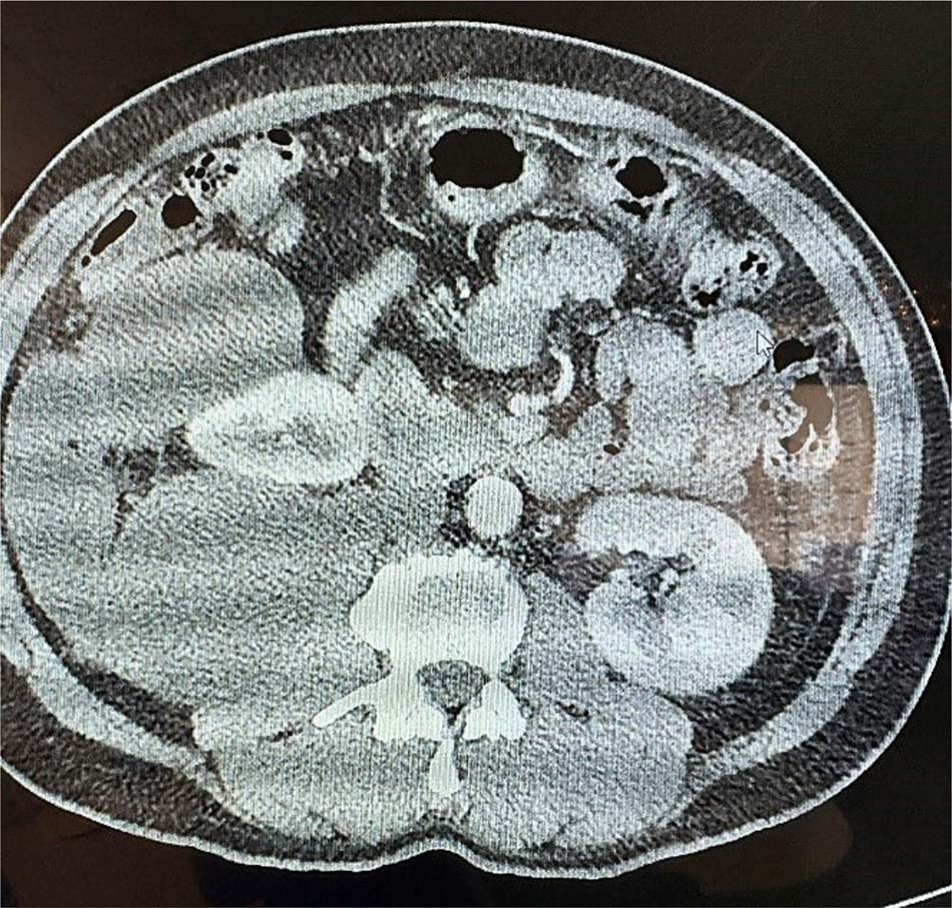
Transverse CT of the mucinous adenocarcinoma.
